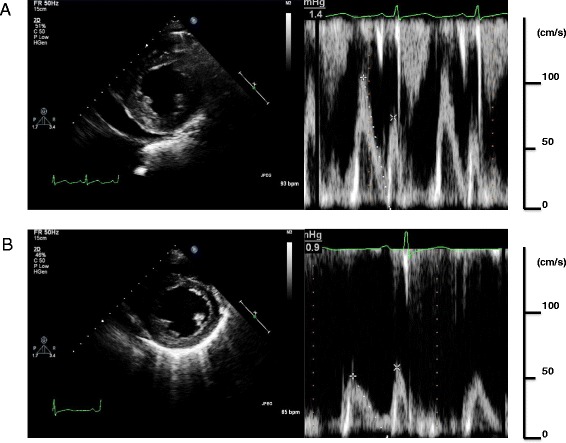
A transthoracic echocardiogram showed a moderate amount of pericardial effusion. A pseudonormal pattern of transmitral flow was observed on administration of thiamine (a). Two days after thiamine administration, pericardial effusion decreased and the mitral Doppler findings showed that constrictive physiology was resolved after thiamine replacement therapy (b)
